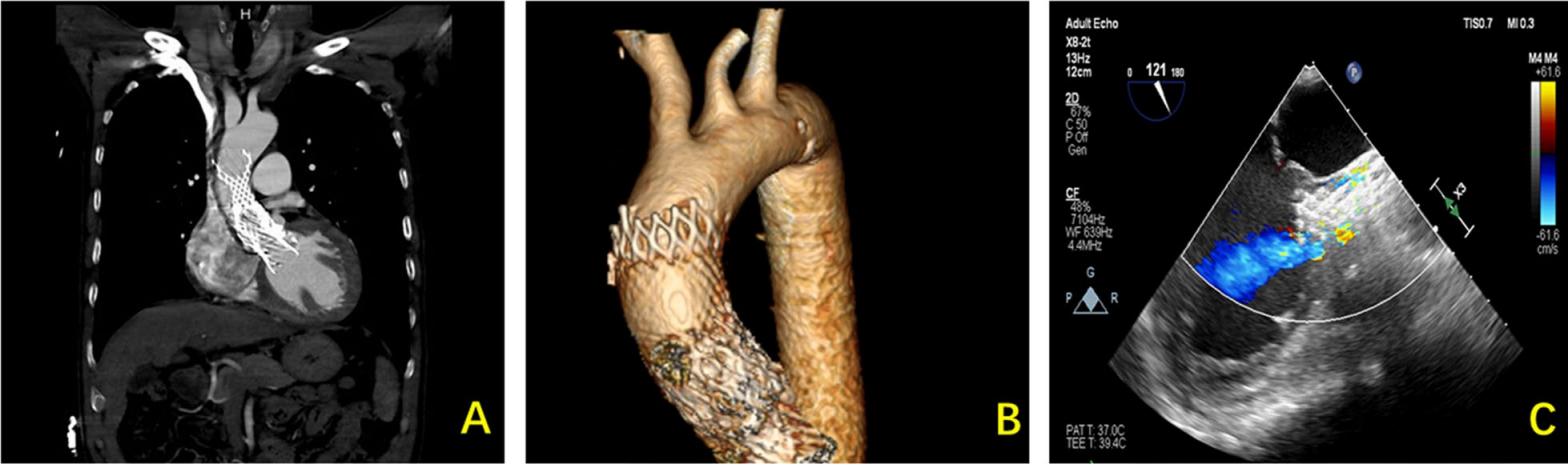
Preoperative Images of TAVR prosthesis migration in a male patient who underwent a previous TAVR procedure with four bioprostheses. A High density shadows of the devices were overlapping inside the ascending aorta. B The crown frame of the upper device was identified to protrude outside of the aortic wall. C A severe paravalvular leakage was identified by preoperative transesophageal echocardiography
